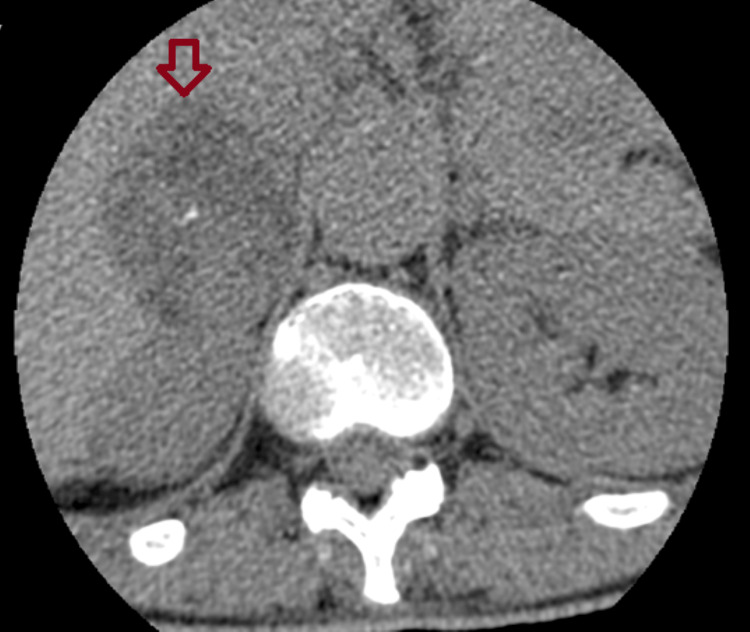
Adrenal imaging findings of Patient 2 An abdominal CT (transverse plane) of a 51-year-old woman showing a right adrenal incidentaloma measuring 46 × 43 mm.
